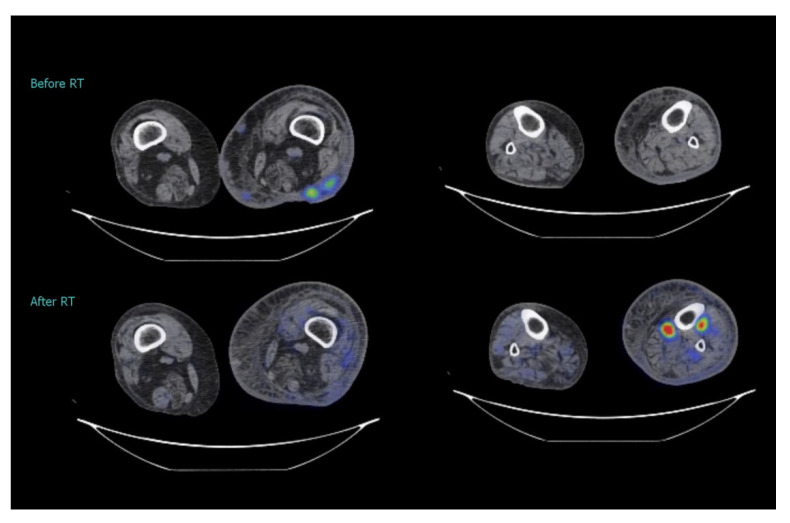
Comparison between 18F-FDG PET images: three months after radiotherapy several 18F-FDG-avid nodules appeared at the interface between muscles and bones. 18F-FDG PET, fluorodeoxyglucose positron emission tomography.
